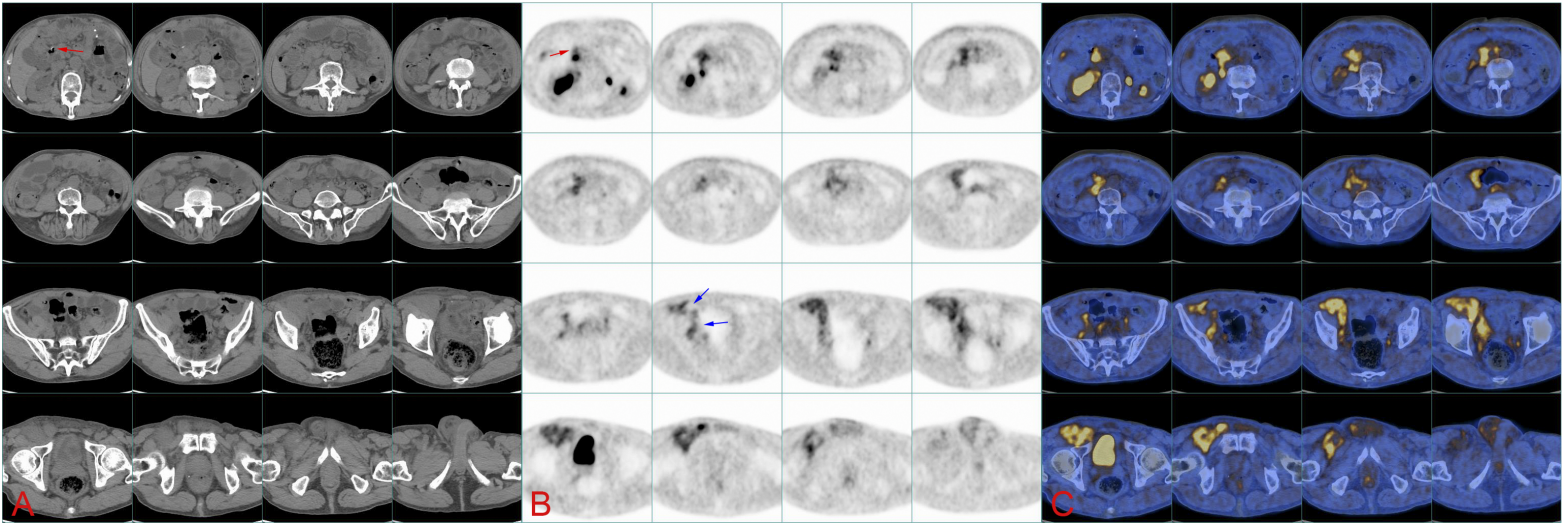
(A) Continuous CT images of the abdomen show the location of soft tissue on the right pelvic wall and groin, while the image of tumor in the abdomen is not clear. Red arrows point at the duodenal stump. (B)
